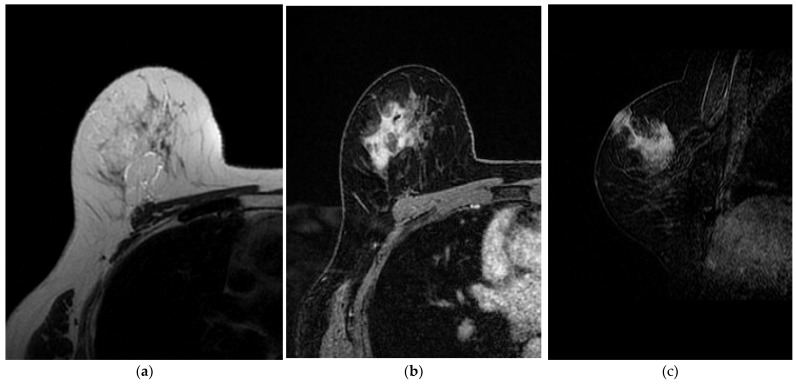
A 60-year-old patient with PBA. (a) T2-weighted, (b) axial 3D gradient echo T1-weighted post-contrast and (c) sagittal 3D gradient echo T1-weighted post-contrast images show an irregular mass, with non-circumscribed margins, characterized by heterogeneous enhancement, with skin thickening and invasion.
